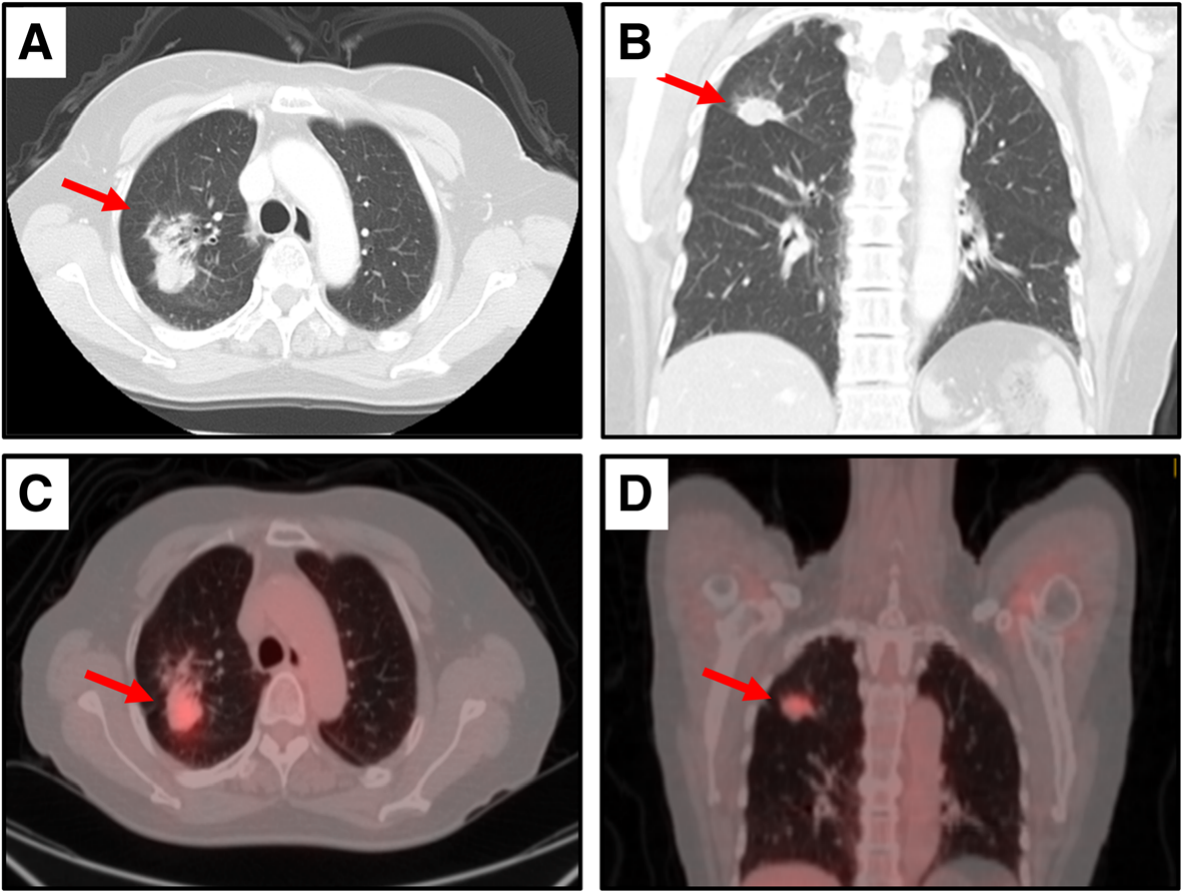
Imaging findings for patient. a, b Chest computed tomography scan revealed an irregular tumor in the right upper lobe, which tumor was suspected to mean lung cancer (arrow). c, d Positron emission tomography revealed high levels of accumulation of ^18^F-fludeoxyglucose in the right upper lobe (arrow) (maximum standardized uptake value of 4.09)
